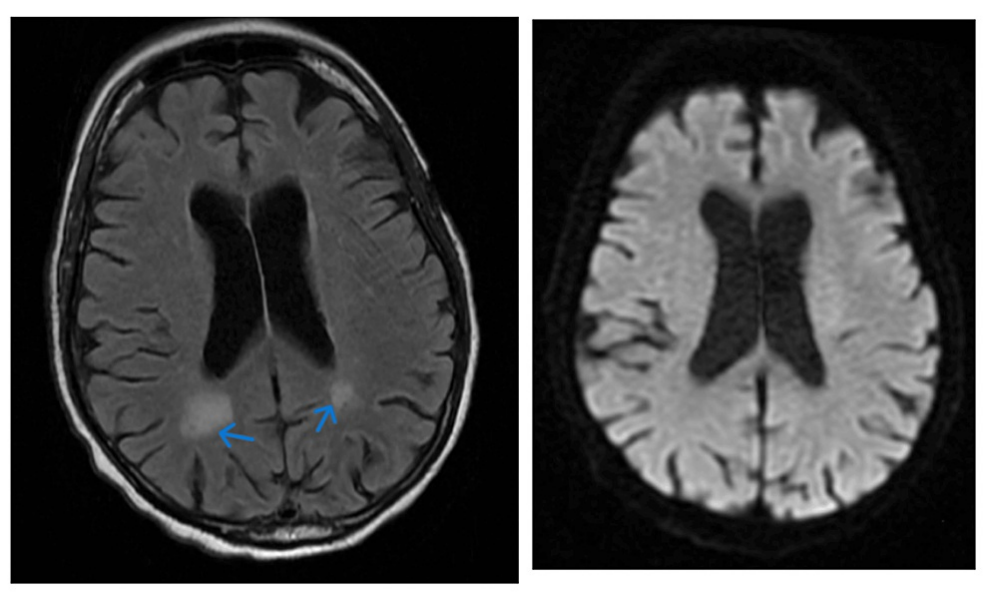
MRI T2 FLAIR images (blue arrow left) demonstrate areas of hyperintensity in the periatrial white matter bilaterally. There is no signal abnormality within the corpus callosum or along the callosal septal interface. DWI image (right) demonstrates no evidence of enhancing parenchymal lesion or abnormal leptomeningeal enhancement and shows mild to moderate cerebral atrophy and chronic microvascular ischemic changes. MRI: Magnetic resonance imaging, T2 FLAIR: T2 and fluid-attenuated inversion recovery
